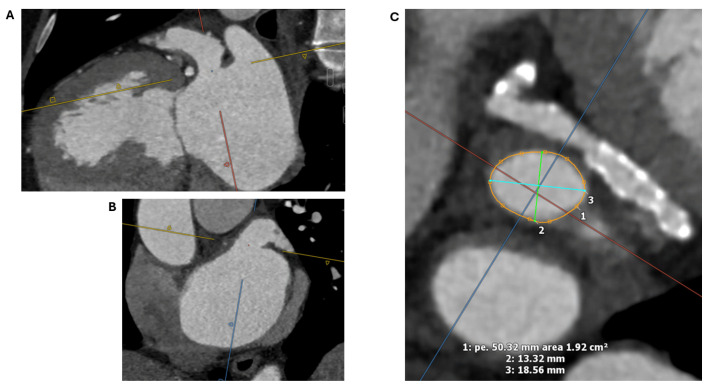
Cardiac CT measurement of the LAA ostium. Using 3D multiplanar reconstruction, cutting a double oblique plane across the coumadin ridge and the left circumflex artery (A,B) creates an en-face view of the ostium (C), determining its shape, perimeter (1), minimum (2) and maximum (3) diameters, as well as area (1). CT: Computed tomography; LAA: Left atrial appendage; 3D: Three dimensional.
